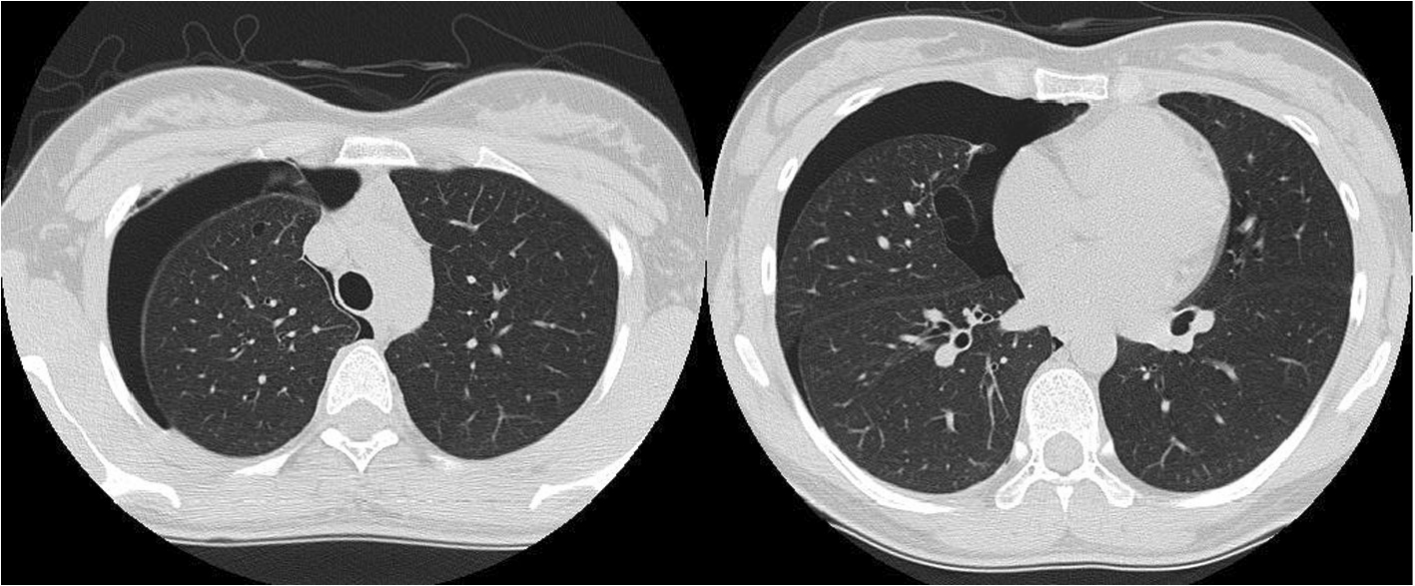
Chest CT showed right-sided pneumothorax and multiple cystic lesions in both of the patient's lungs. Adhesion and thickened visceral pleura were found.
